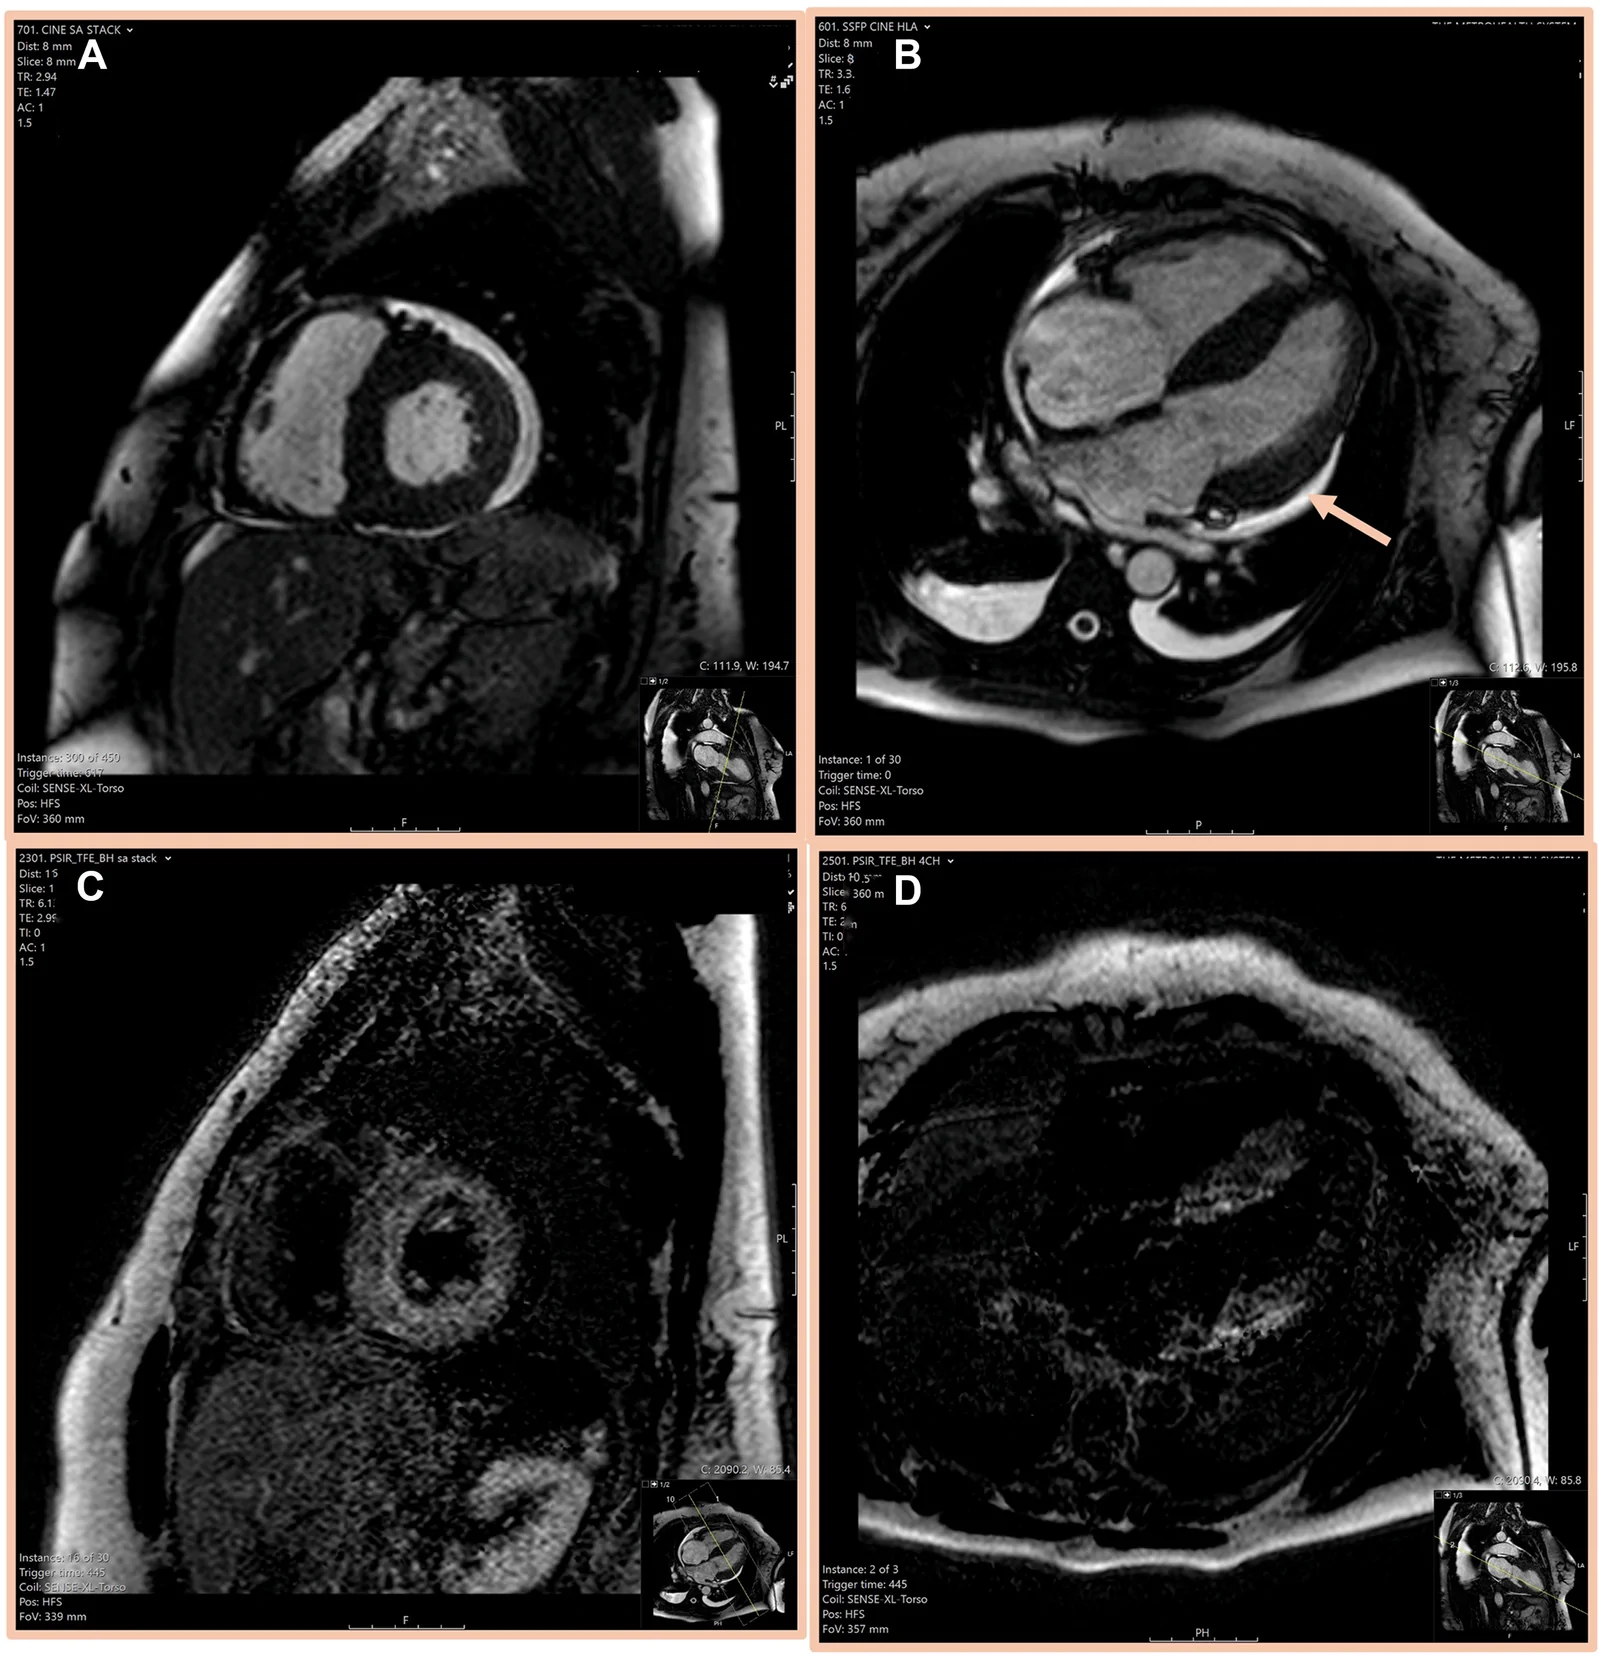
Cardiac Magnetic Resonance Image (A) Short-axis basal left ventricular steady-state free precession cine image in end diastole. (B) Four-chamber steady-state free precession cine image in end diastole, demonstrating biventricular wall thickening and biatrial enlargement. (C and D) Phase-sensitive inversion recovery postgadolinium images with gadolinium uptake in the myocardium, particularly the basal and mid–left ventricular segments (image D), showing absence of gadolinium in the blood pool at an inversion time of 320 ms after a double dose of gadolinium contrast. Images were acquired approximately 10 to 12 minutes after administering gadolinium contrast. These findings are characteristic of cardiac amyloidosis.
